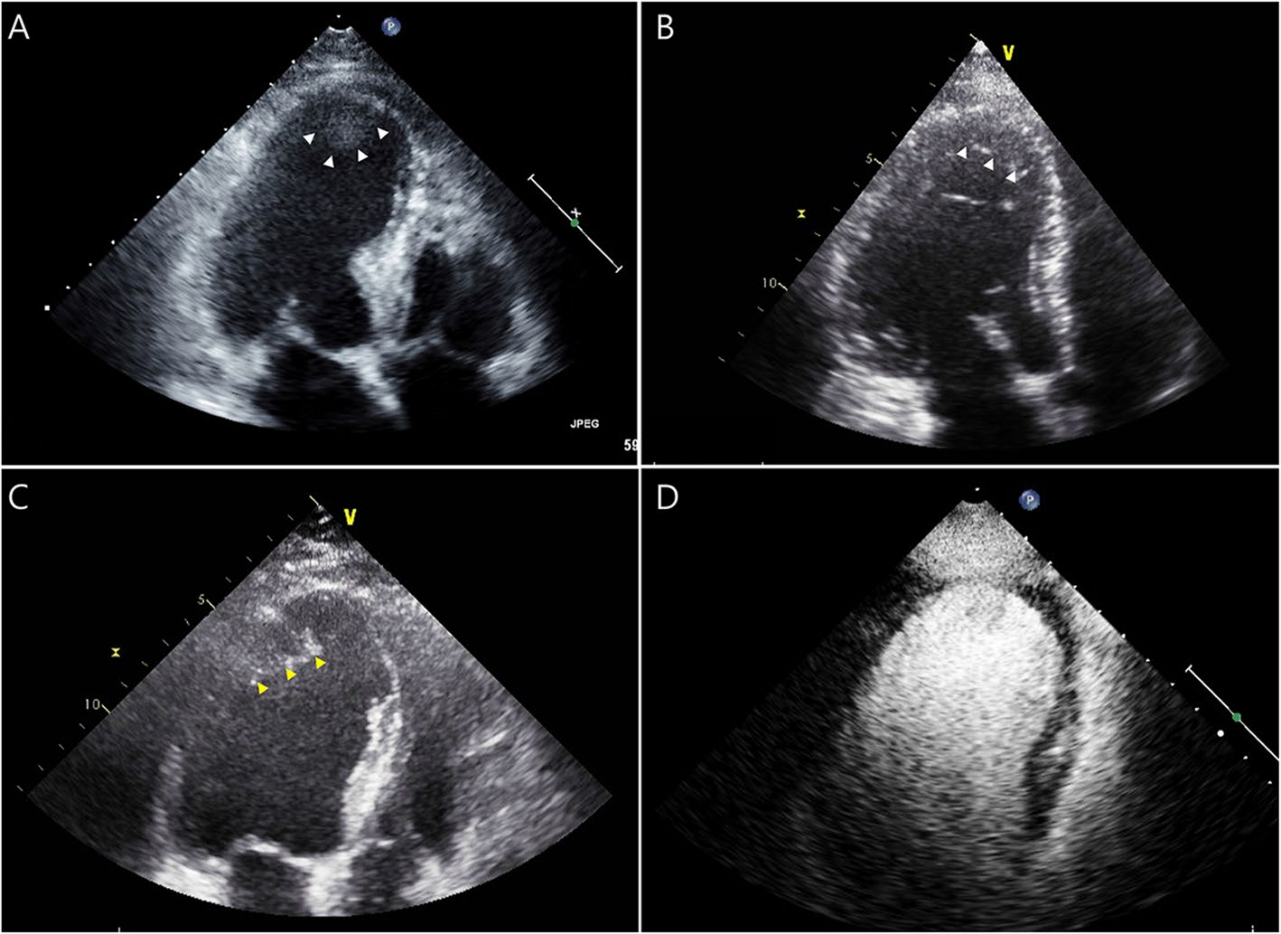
Several examples of suspected left ventricular thrombus. A Apical four-chamber view on conventional echocardiography, showing near-field clutter (white arrow). B Conventional echocardiography displaying left ventricular trabeculation (white arrow). C Suspicion of left ventricular thrombus (yellow arrow) on conventional echocardiography. D Contrast echocardiography demonstrating the exclusion of left ventricular thrombus
